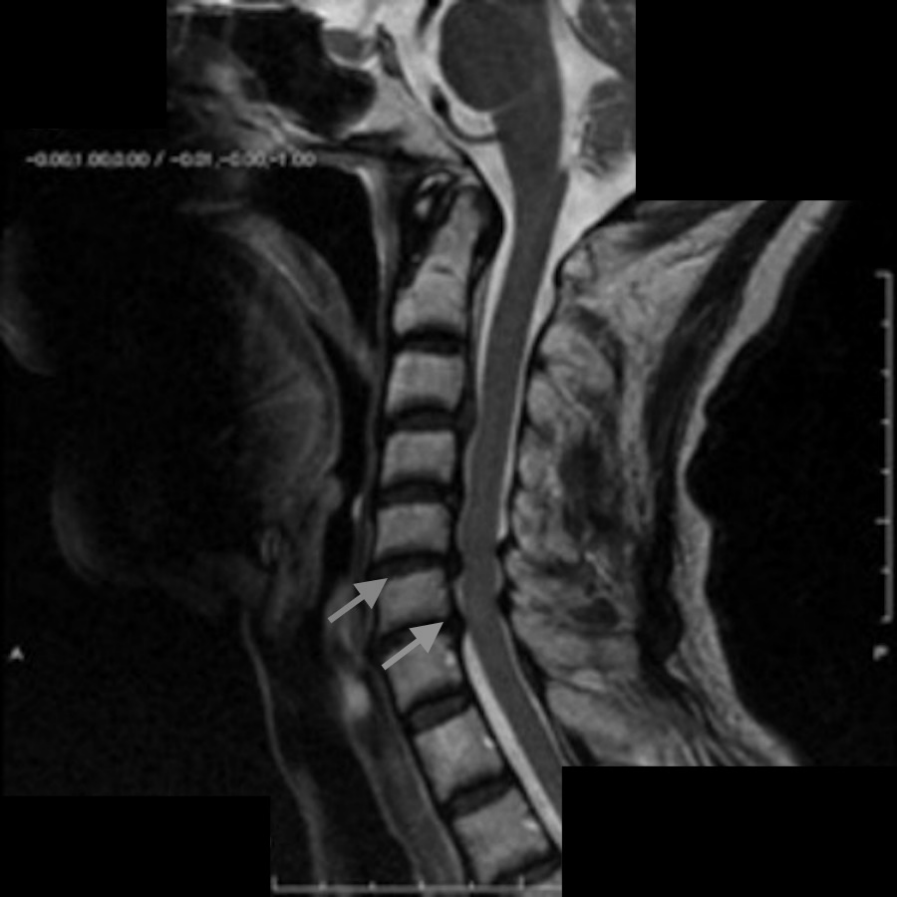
MRI showing dilated biliary ducts (white arrow) with a tapering of the distal common bile duct (yellow arrow) Cervical disc herniation at the level of C3–4 and C4–5 (gray arrows)
